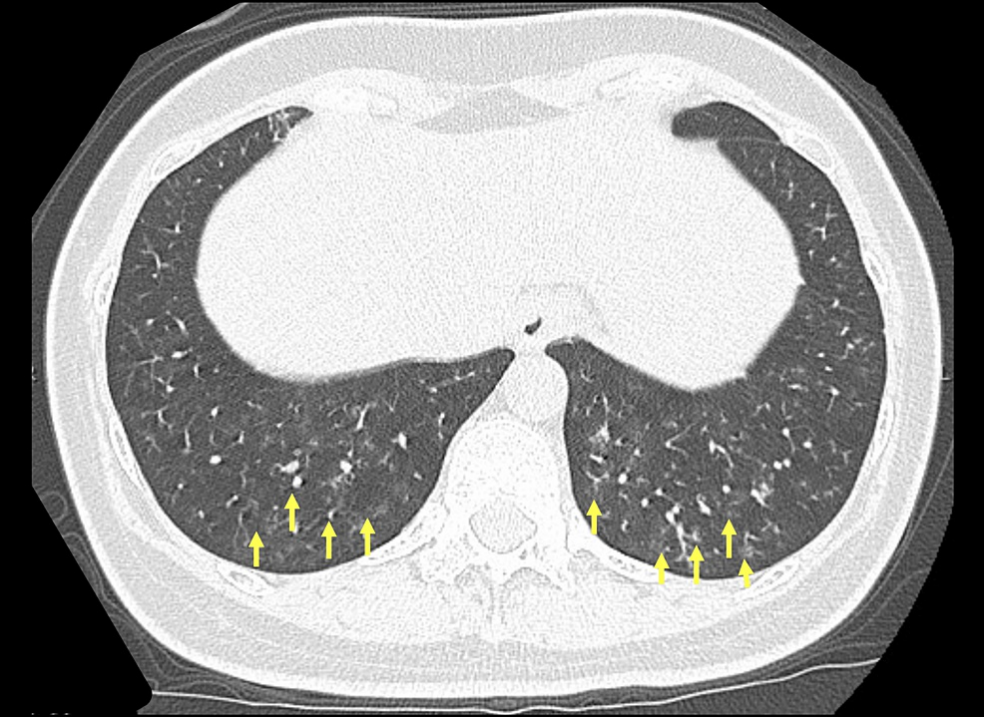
Chest computed tomography showing bilateral diffuse lobular central pale granular shadows.
